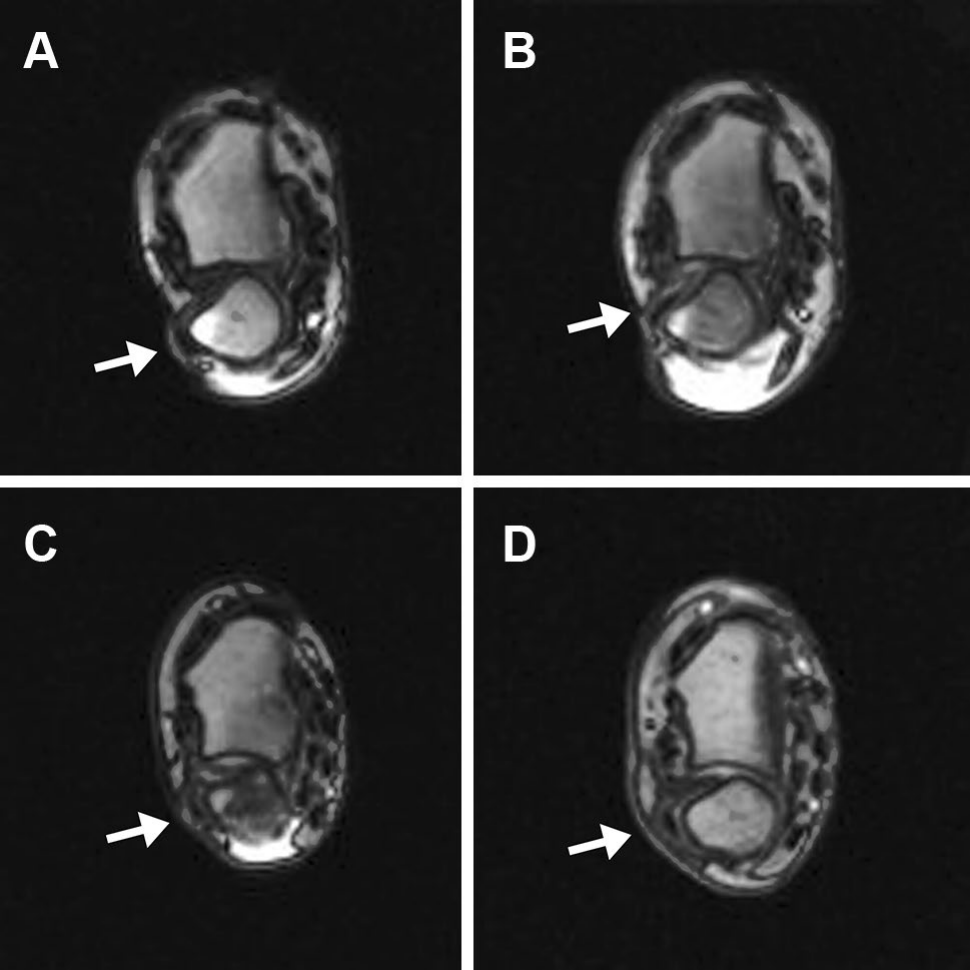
Rotation-movie MRI visualizing ECU tendon displacement (arrow) in full supination: ECU tendon dislocation before surgery (a) and corrected ECU position postoperatively without dislocation (b); ECU tendon dislocation before surgery (c) and persisting ECU tendon dislocation postoperatively (d)
